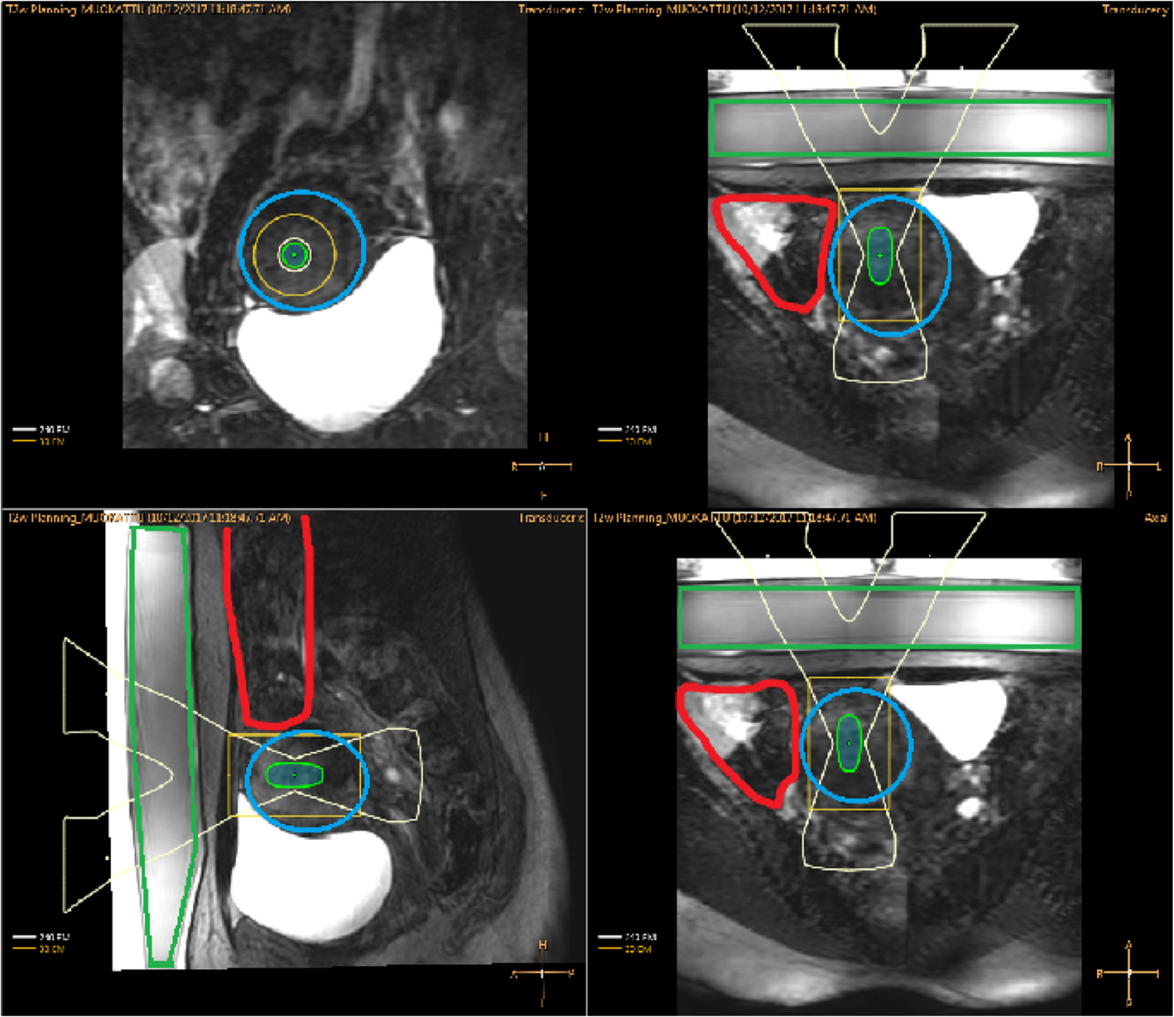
T2W planning images displayed on the therapy planning console with ultrasound beam view after application of wedged gel pad where red color boundary is bowels, blue is uterine fibroid, and green is the gel pad
